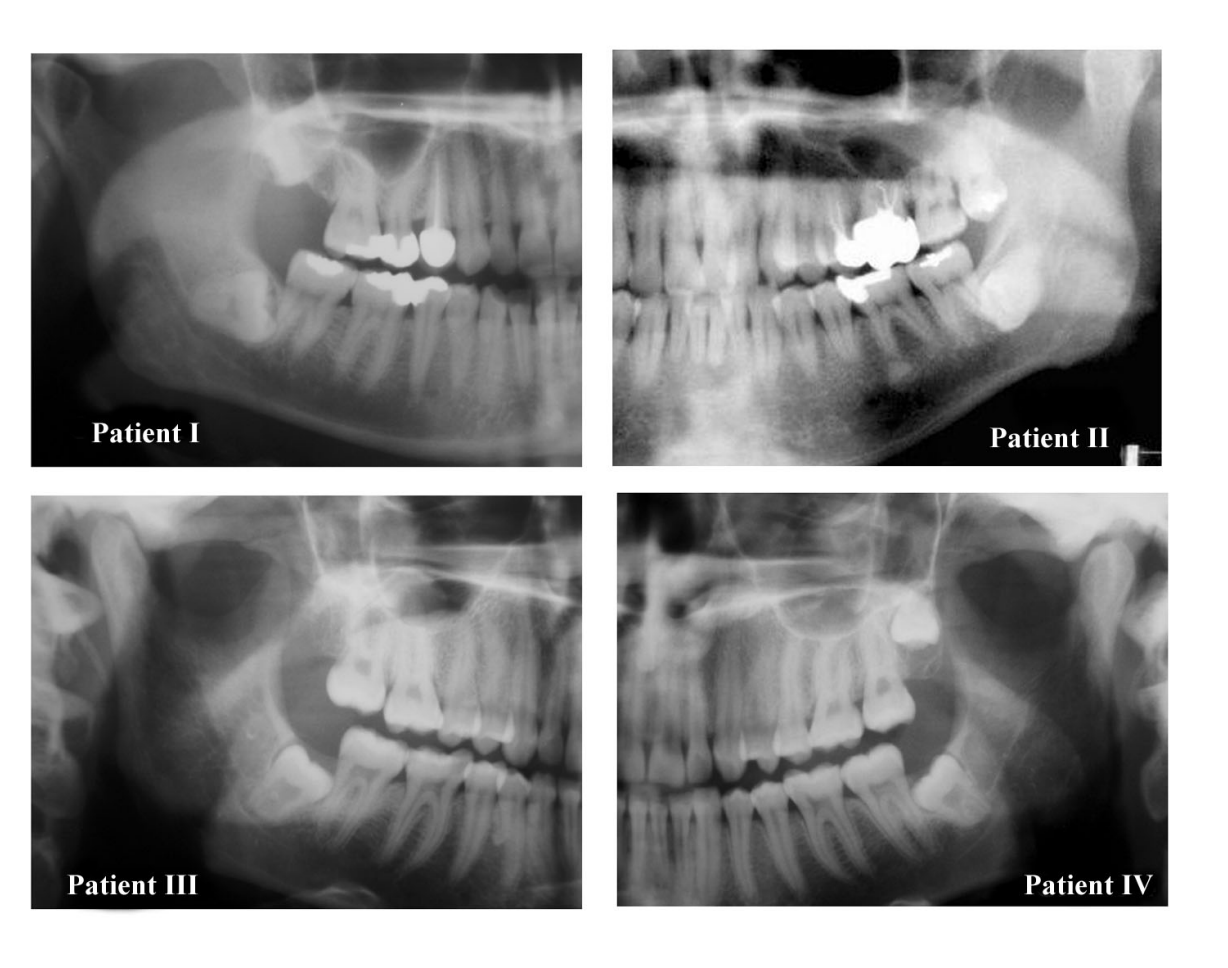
A montage of the panoramic radiographs of the four patients showing in each case the close spatial relationship between the mandibular third molar teeth and the IAN.
